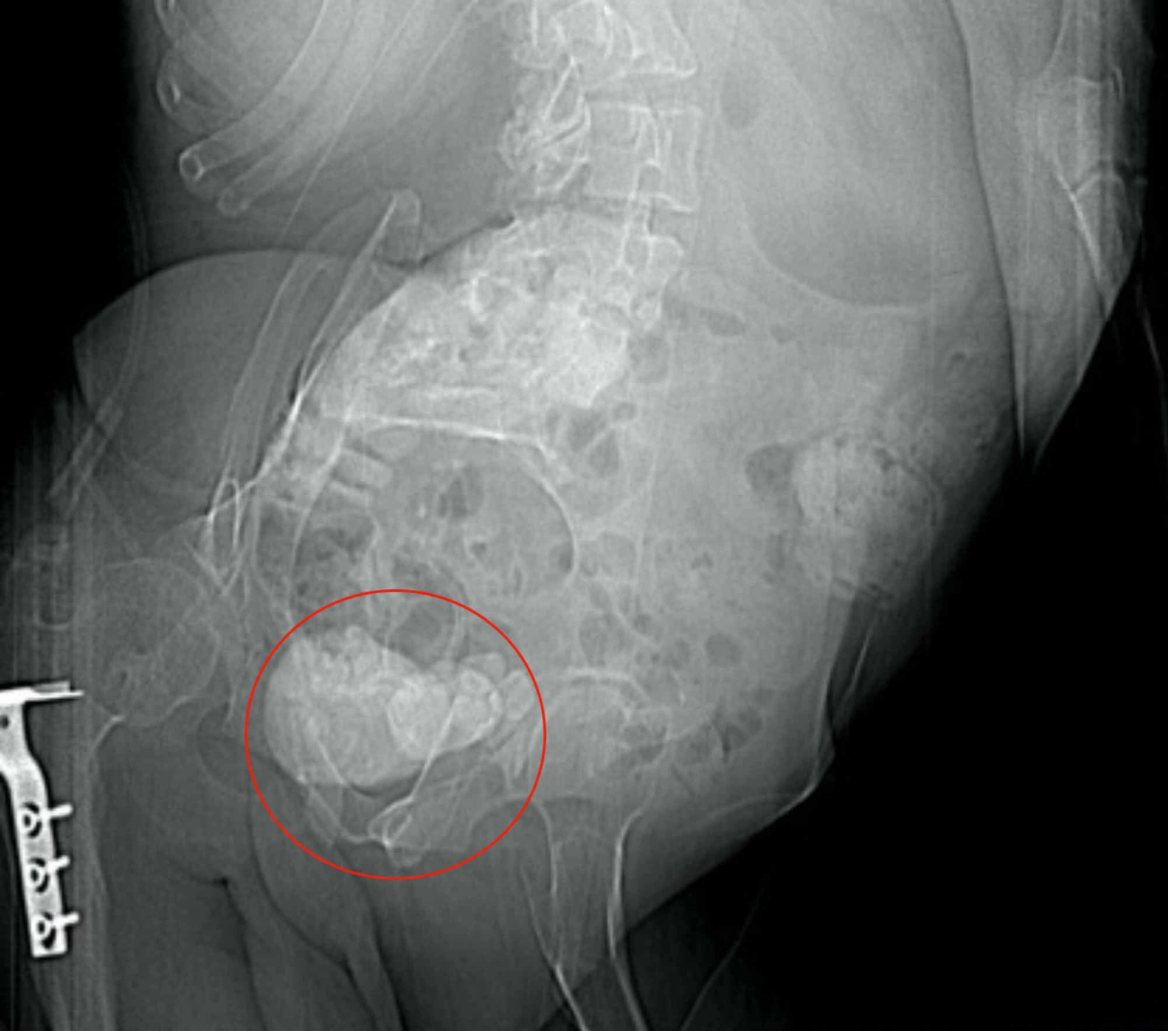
Plain X-ray KUB shows large stone burden in the urinary bladder and the prostate gland KUB: kidneys, ureters and bladder
